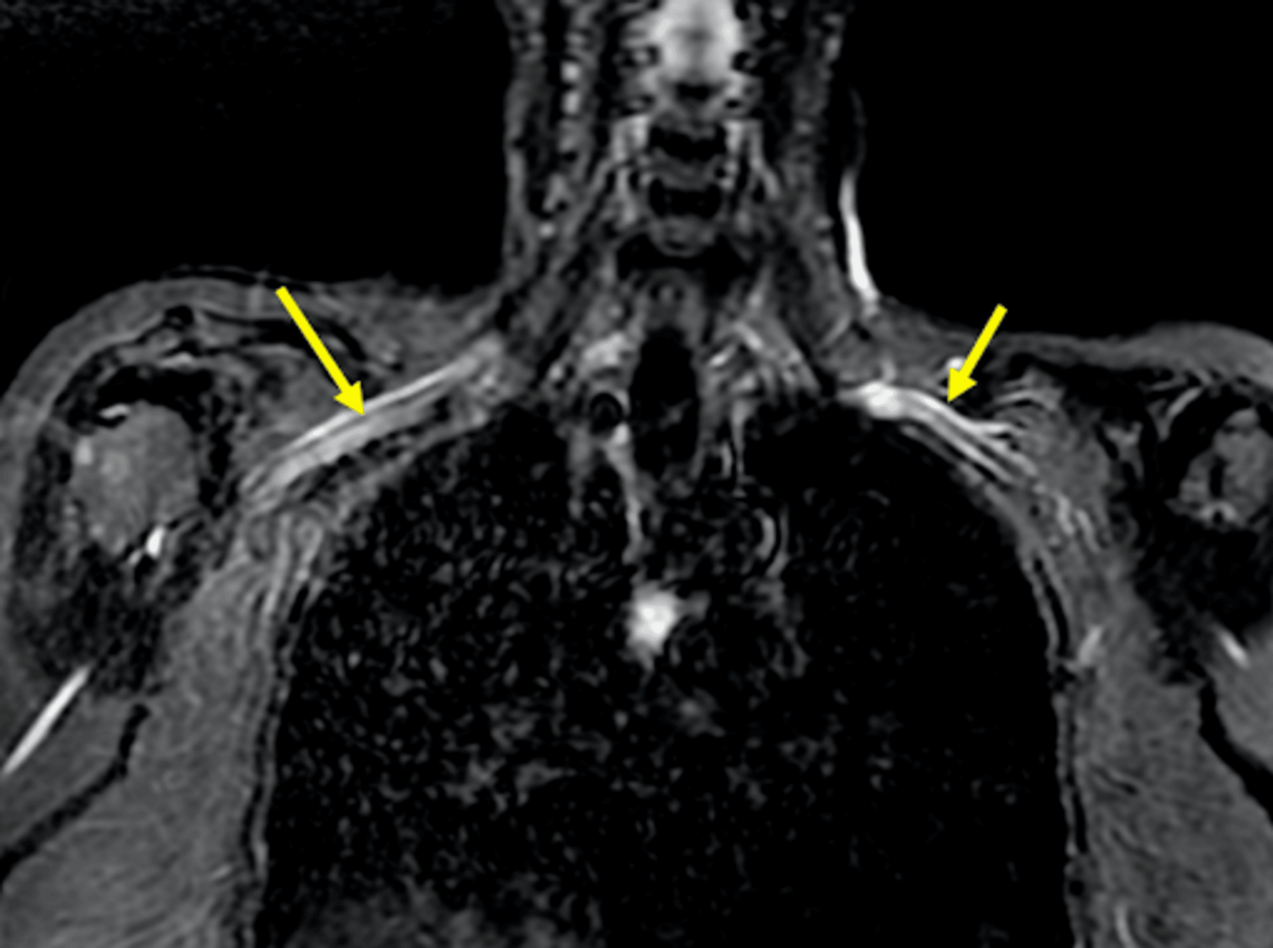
An MRI scan showing signs of brachial plexus neuritis with bilateral edema The yellow arrows indicate areas of bilateral edema, which are represented by a hypersignal on the short tau inversion recovery (STIR) sequence.
